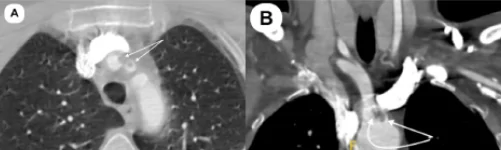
Computed tomography angiogram of the head and neck, (A) axial view and (B) coronal view, demonstrating a free-floating thrombus (arrows) at the junction of the left common carotid artery and brachiocephalic trunk.
